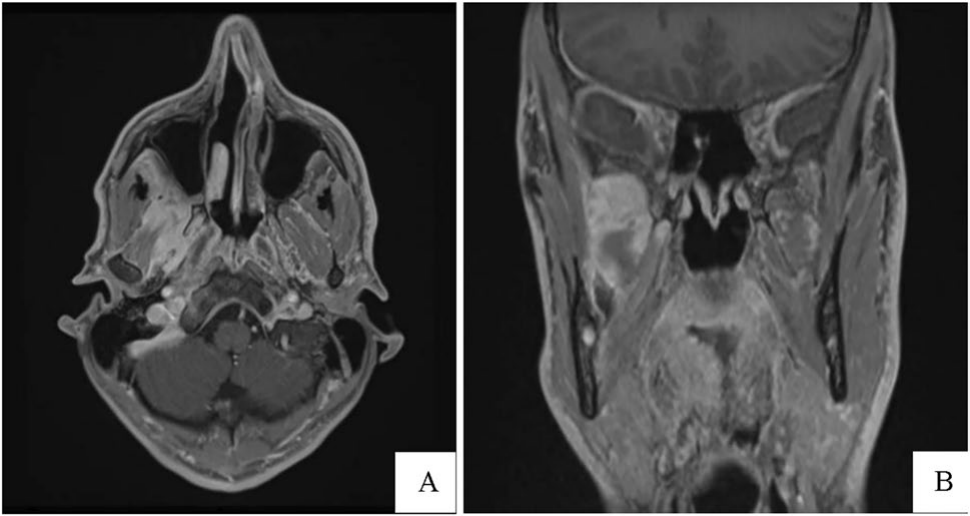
MRI axial (A) and coronal plane (B) showed T1W1 contrast enhancement uptake in the pterygopalatine fossa, extending medially to the sphenopalatine foramen and laterally to the infratemporal and masticatory space
